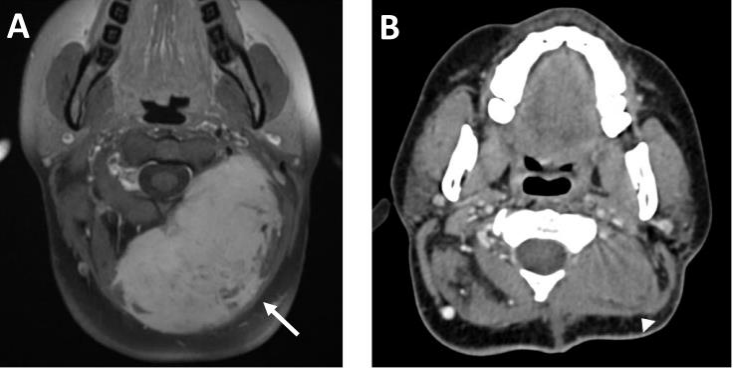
Swelling in the Left Posterior Neck Region of a 42-year-old Female Patient for Two Years. A: Pre-surgery, axial post contrast MRI of neck shows heterogeneously enhancing solid lesion reaching up to the C1 vertebra abutting the spinal epidural space on left (arrow, A). B: Post surgery and radiotherapy, axial CT showing significant decrease in the size of the lesion (arrowhead, B).
